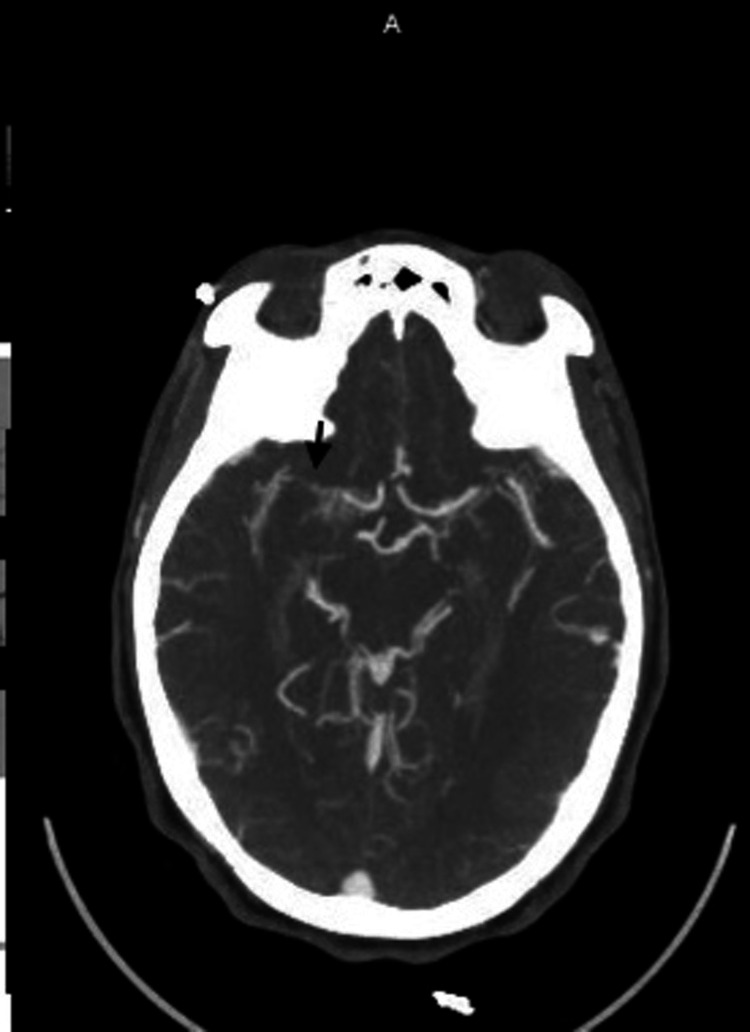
Computed tomography angiography of the head showing stenotic lesion within the horizontal segment of the right MCA MCA, middle cerebral artery
